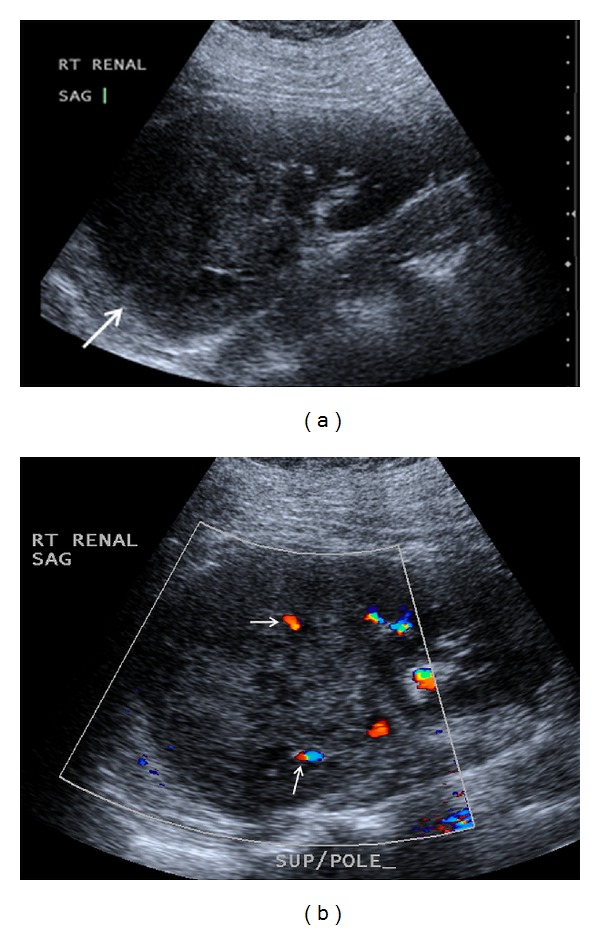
(a) Sagittal ultrasound image of the right kidney shows a large mass replacing the upper pole of the right kidney (arrow). The echotexture is similar to renal parenchyma with mild internal heterogeneity and a few cystic spaces. (b) Sagittal ultrasound image with Doppler shows internal vascularity (arrows) within the large mass replacing the superior pole of the right kidney.
